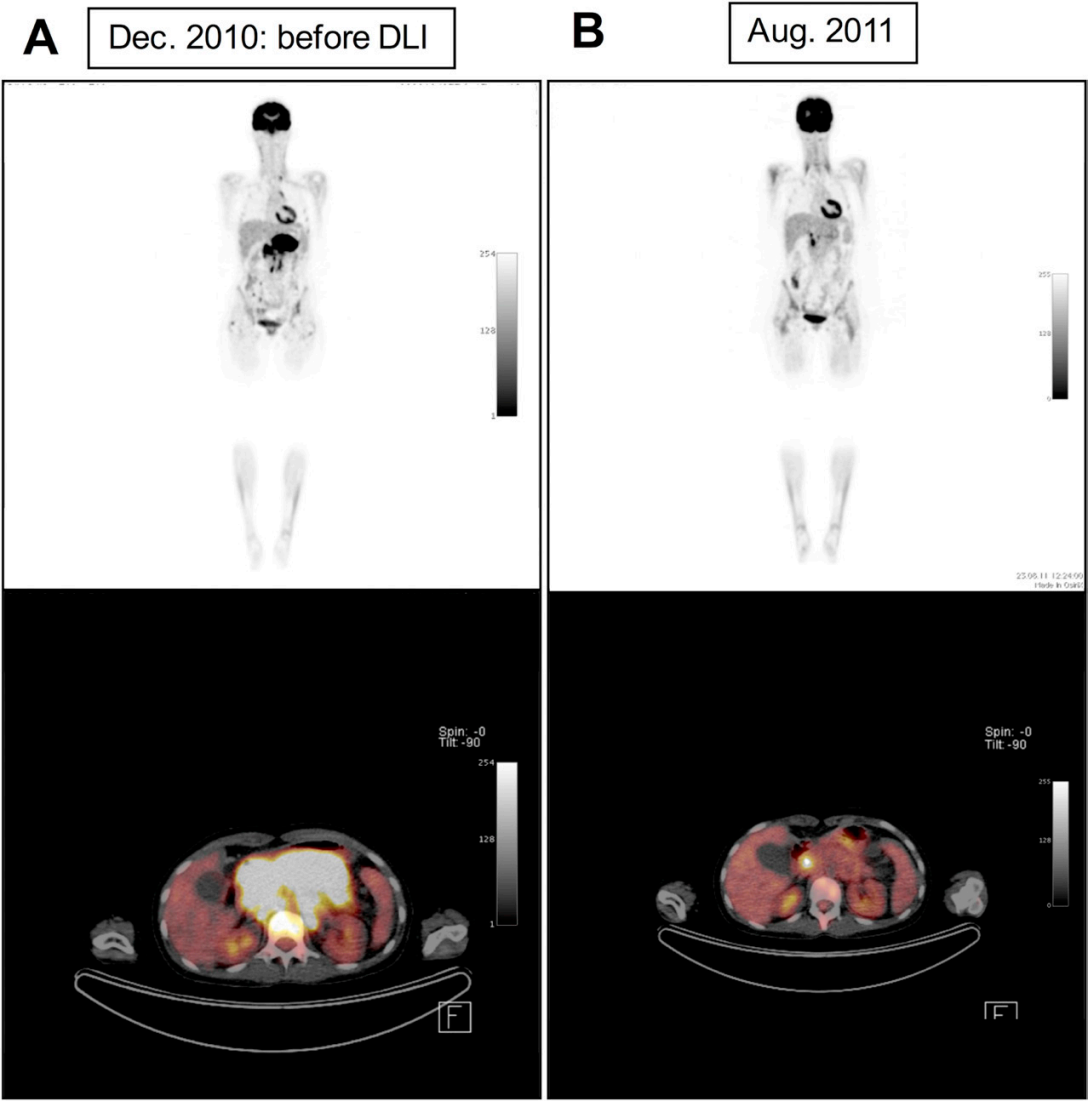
Positron emission tomography–computed tomography (PET-CT) of patient RMS#4 shows reduction of pathological [^18^F] fluorodeoxyglucose (FDG)-uptake of pancreatic tumour mass under combinatory treatment including DLI, hyperthermia and mini-ICE chemotherapy indicating partial tumor regression (A: before DLI, B: eight months after DLI).
